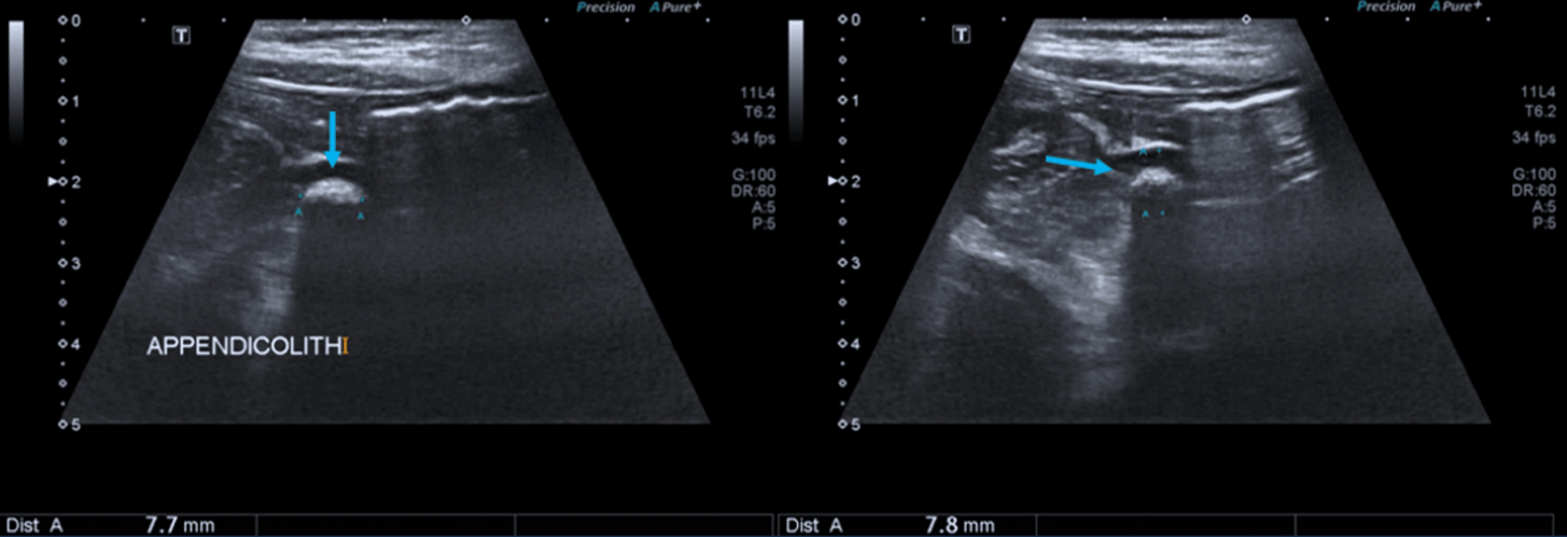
Ultrasound images show an appendicolith with distal acoustic shadowing at the base of the inflamed appendix measuring 7.8 mm
